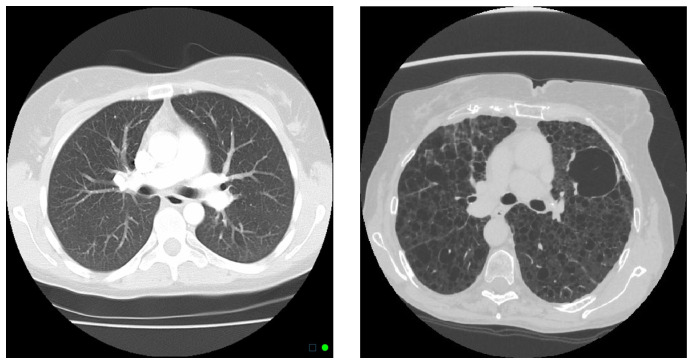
Comparing the thoracic CT scan of healthy lungs (left) and lungs with LAM (right). In the image on the right, round, air-filled cysts of a range of sizes appear as dark voids in the lung tissue.
